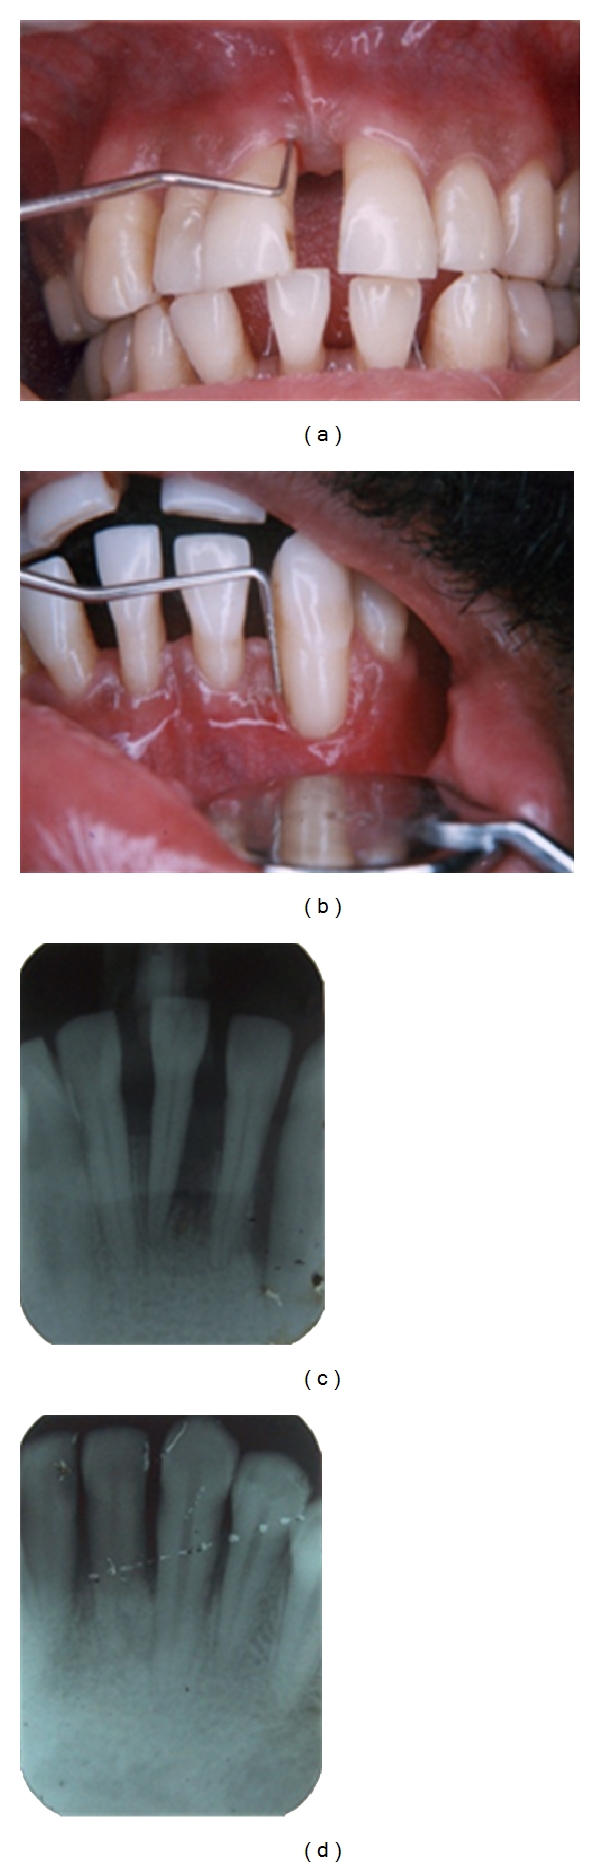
(a) and (b) Postoperative photographs showing probing depths within normal limits with excellent gingival condition; however, there was slight increase in recession after healing. (c) and (d) Comparison of preoperative and 1 year postoperative radiographs shows good bone fill at the defect in relation to 33.
